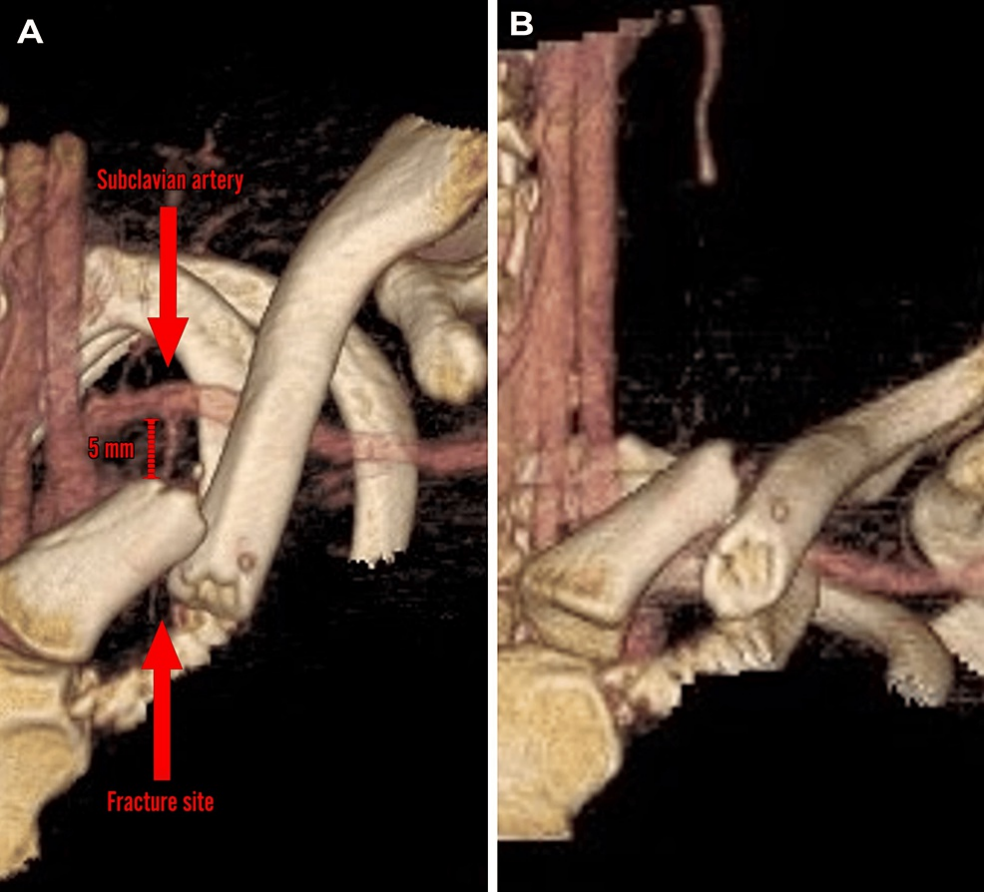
CT angiography of the left upper limb showing the fracture in close proximity to the subclavian artery (approximately 5 mm posterior to the medial fragment) Figure A shows the fracture in cephalo-caudal view where the medial fragment is displaced posteriorly. Figure B shows the fracture in anterior-posterior view where the medial fragment is displaced superiorly
